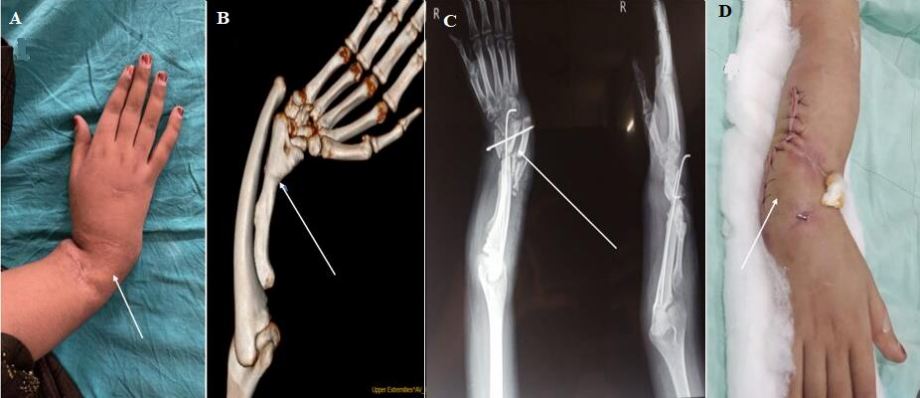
A) gross deformity of right forearm with prominent ulnar head; B) 3D-computed tomography image showing deformed ulna with radio ulnar dislocation with short radius; C) post operative X-ray showing fixation of deformity by k wire in situ; D) correction of forearm angulation deformity
